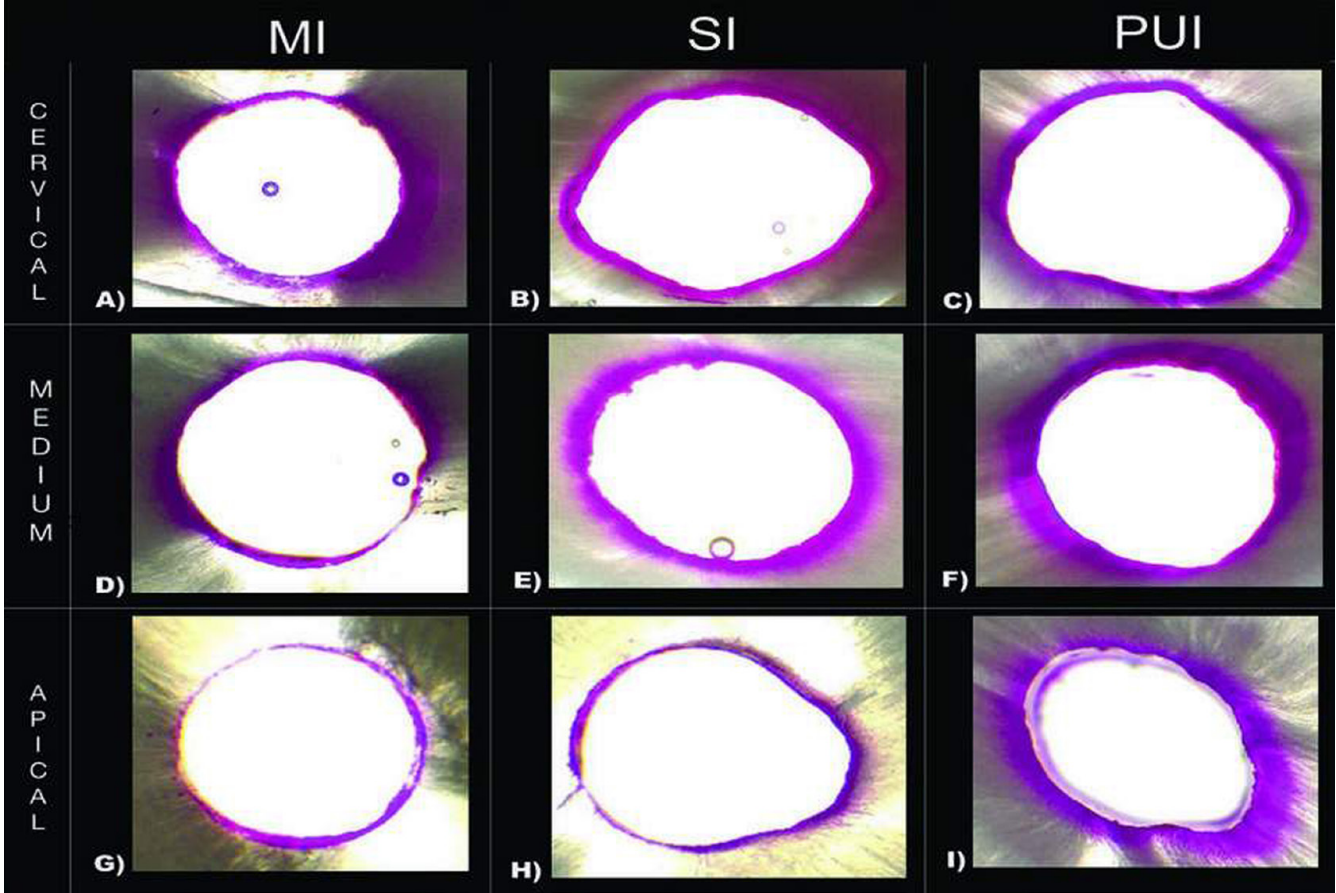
Different irrigation methods at different levels: A) Manual Irrigation Cervical B) Sonic Irrigation Cervical C) Passive Ultrasonic Irrigation Cervical D) Manual Irrigation Medium E) Sonic Irrigation Medium F) Passive Ultrasonic Irrigation Medium G) Manual Irrigation Apical H) Sonic Irrigation Apical I) Passive Ultrasonic Irrigation Apical.
